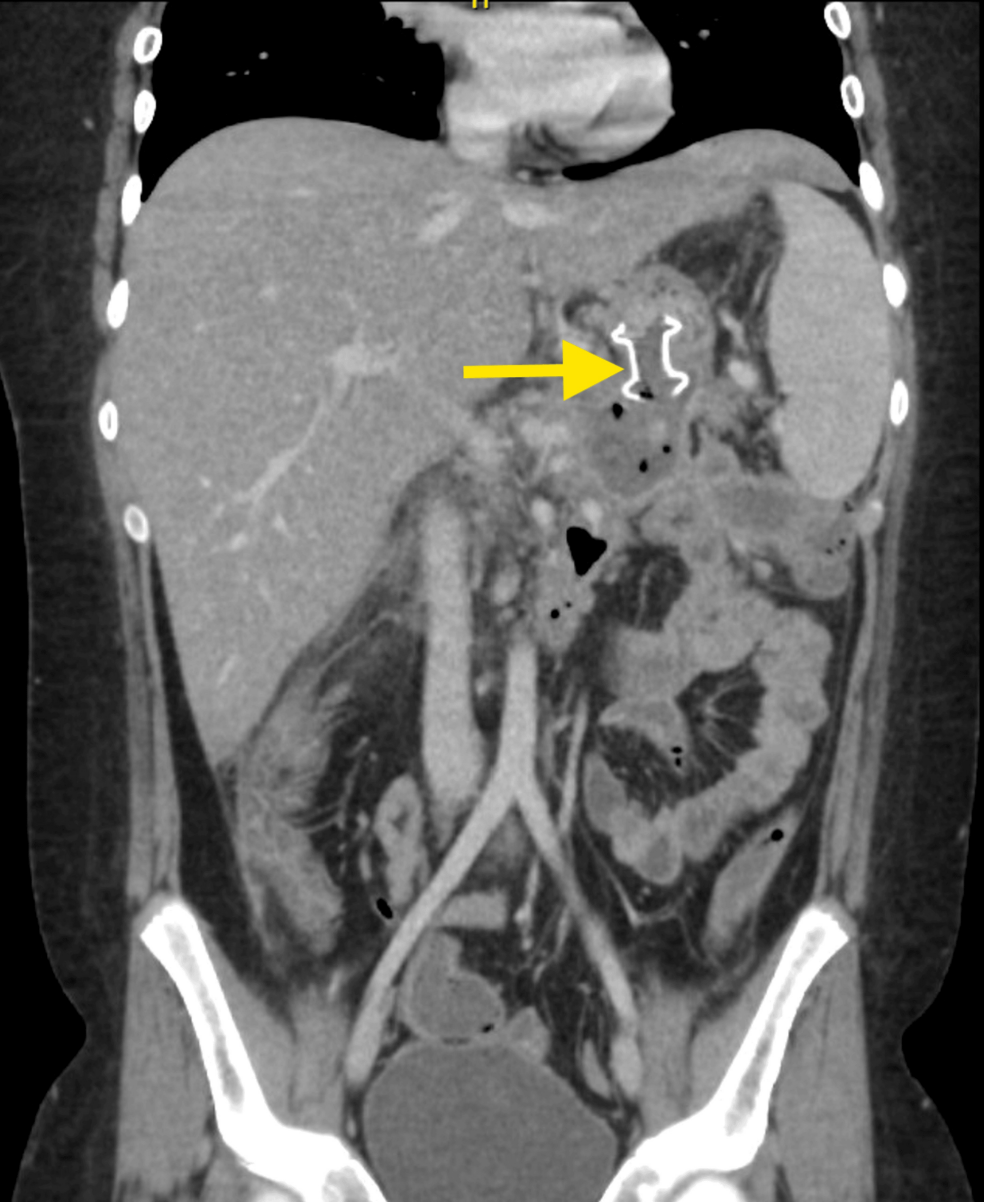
Axial contrast-enhanced CT abdomen image showing obstructed LAMS LAMS: lumen-apposing metal stent
